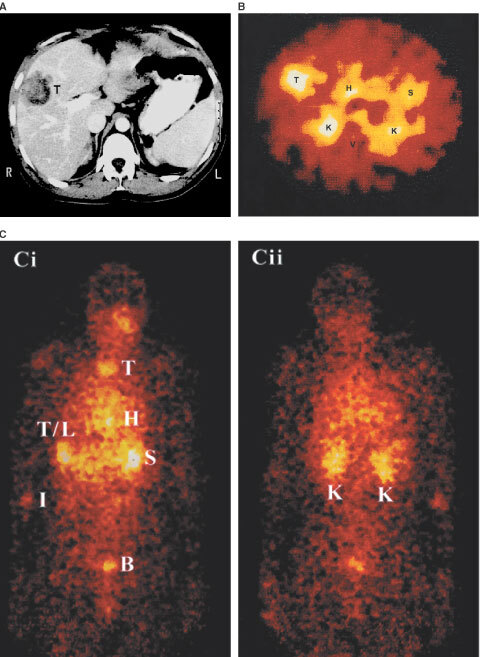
Imaging results for patient 5 24 h after injection of ^131^I-hDFM. (A) CT scan through the liver of where a liver metastasis is arrowed, (B) corresponding gamma camera cross-sectional SPECT image showing localisation of ^131^I-hDFM to the liver metastasis and (C) corresponding whole body image (i) anterior and (ii) posterior views. High amounts of radioactivity are shown in areas of intense colour (white–yellow). T/L=tumour/liver, H=heart, K=kidney, S=stomach, V=vertebra, B=bladder, I=injection site, T=thyroid.
